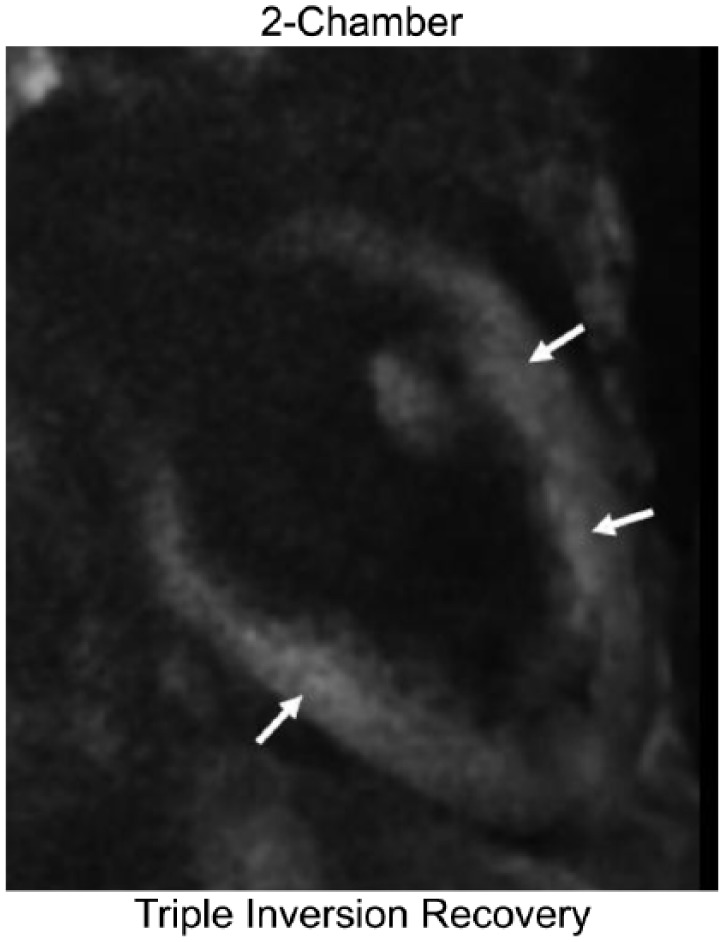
Cardiac magnetic resonance imaging triple-inversion recovery sequence demonstrating hyperenhancement consistent with myocardial edema (arrows).
